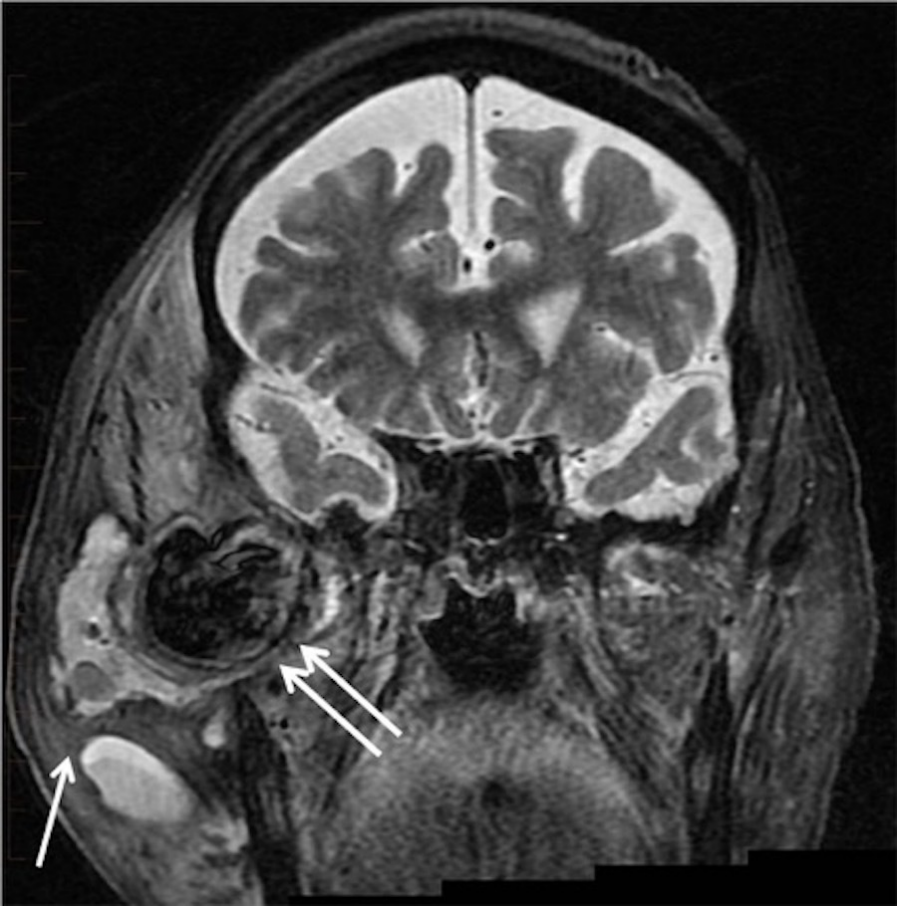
Coronal short tau inversion recovery magnetic resonance imaging reveals a multiloculated, hyperintense fluid collection in the soft tissue lateral to the mandible and zygomatic arch (arrow). Note the separate hypointense collection in the masticator space anterior to the mandibular condyle (double arrow). This is turbulent flow within the pseudoaneurysm.
